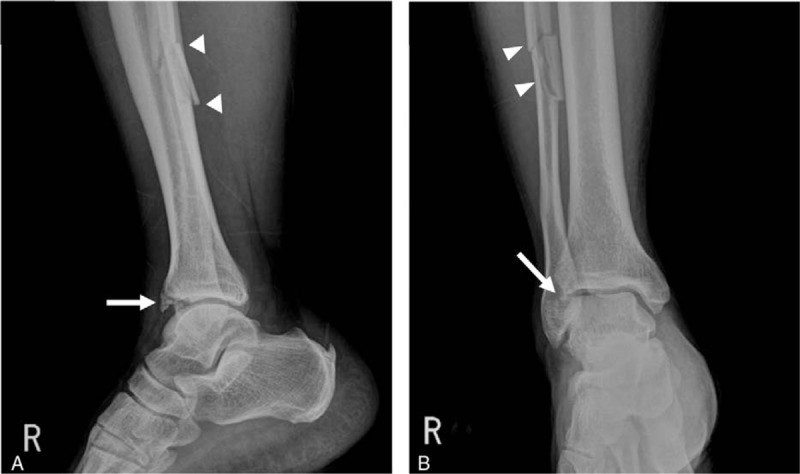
Initial ankle anteroposterior (A) and lateral (B) radiographs demonstrate a segmental fracture of shaft of the right fibula (arrow heads in A and B) and fracture of the anterior lip of the tibial plafond with displacement (arrows in A and B).
